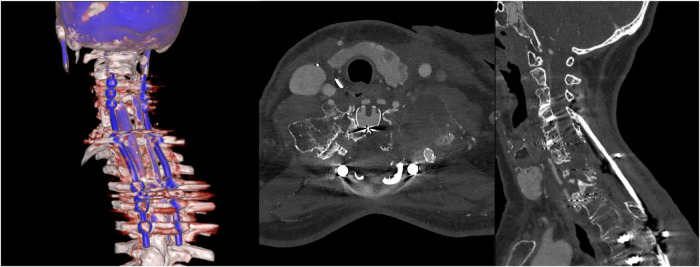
At the 6-month follow-up, a CE photon-counting CT scan shows good local control (with signs of tumor necrosis) and good alignment of the cervicothoracic spine. In the sagittal plane, initial signs of posterior bone fusion are visible between the posterior fibular homoplastic allograft and the vertebral laminae.
